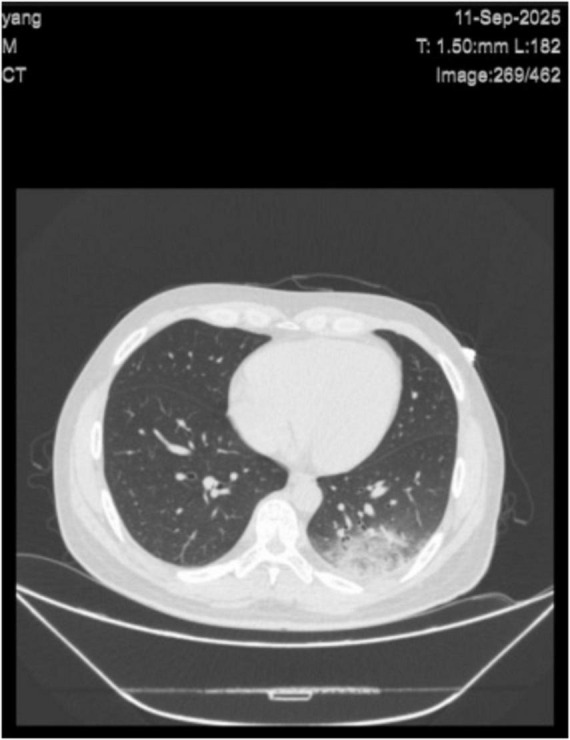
Axial cross-sectional CT scan of the chest shows the lungs, heart, ribs, and part of the spine with normal anatomical structures clearly visualized. Patchy haziness in the left lower lobe is decreased compared with prior images, indicating reduced infection. A tiny amount of left pleural effusion is noted.
